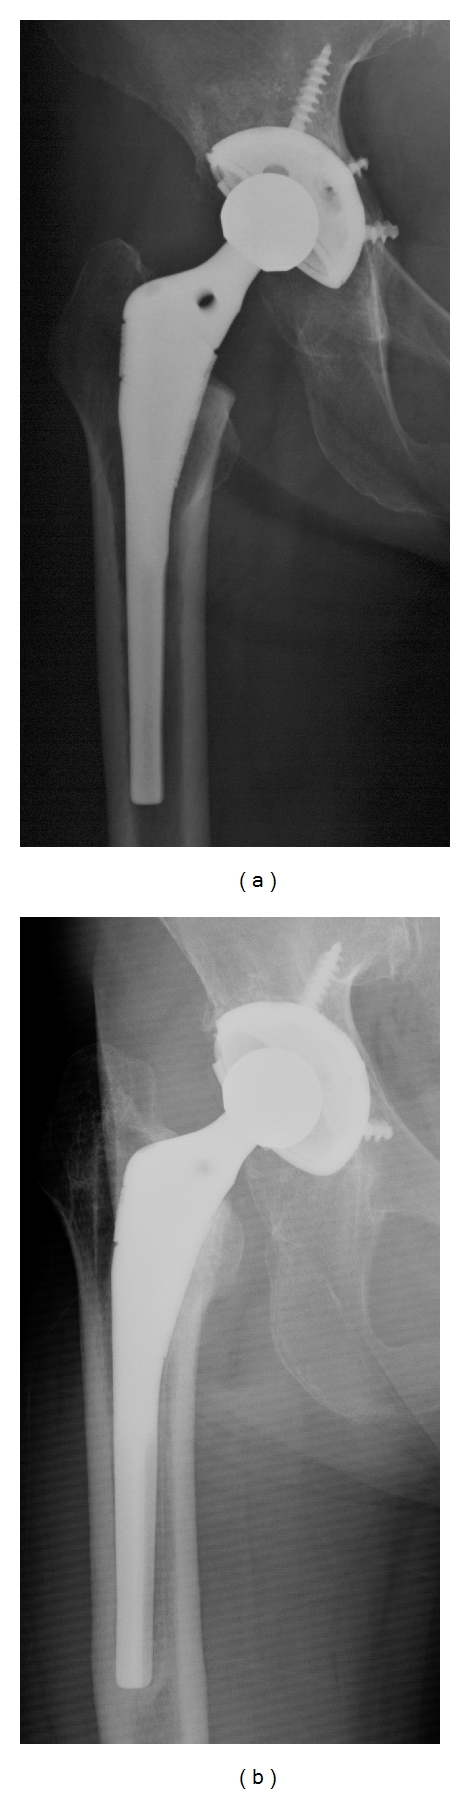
The right hip of 45-year-old female who had received bilateral THA for rapidly destructive coxarthrosis. (a) The postoperative AP radiograph. The stem had been undersized, and metaphyseal fit had been poor. (b) The AP radiograph at 6.1 years after THA. The stem showed subsidence and loosening.
